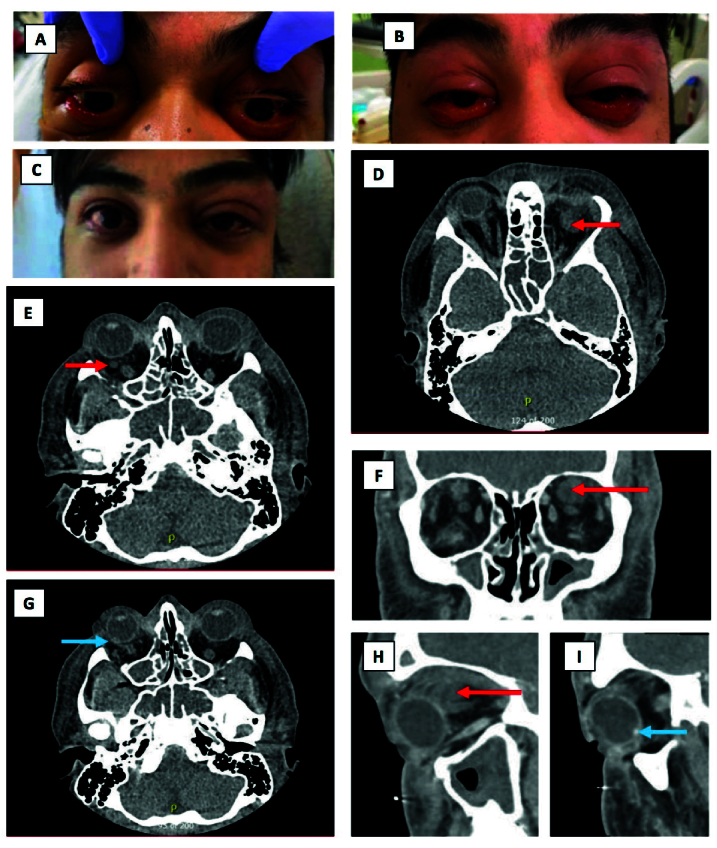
Case 2 (31-year-old male). Photographs showing periorbital edema and conjunctival injection at day 1 (A), day 2 (B), and day 7 of hospitalization (C). CT head and orbit with soft tissue window, axial (D, E, & G), coronal (F), and sagittal (H) sections demonstrating diffuse facial/scalp/periorbital soft tissue thickening and edema with no evidence of orbital abscess. The red arrows show the orbital lesions suspicious for hemorrhage or thrombus, and the blue ones reveal the calcified scleral nodule seen on examination.
